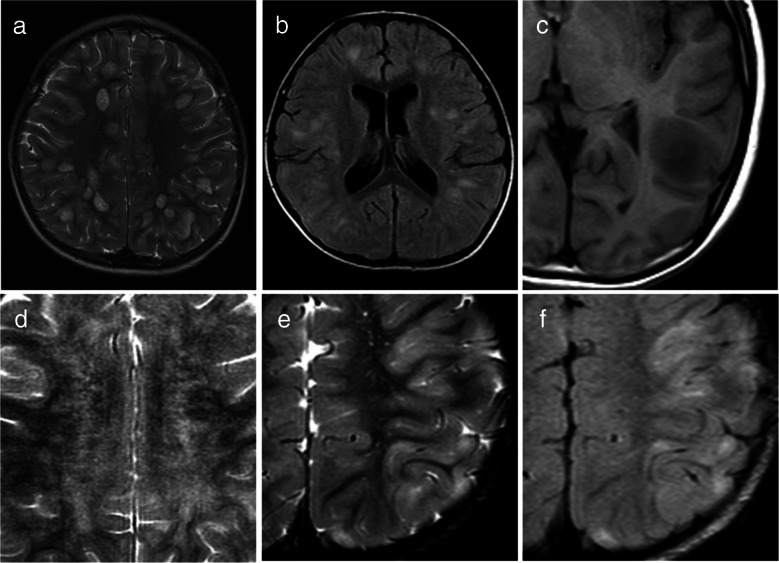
Representative magnetic resonance imaging features considered atypical for acute disseminated encephalomyelitis. a Axial T2-weighted image in a 5-year-old boy with myelin oligodendrocyte glycoprotein antibody-associated acute disseminated encephalomyelitis (MOG-ADEM) shows well-demarcated lesions predominantly involving subcortical white matter. b Axial fluid-attenuated inversion recovery image in a 5-year-old girl with MOG-ADEM shows small (< 2 cm) lesions. c Axial T1-weighted image in an 11-year-old girl with MOG-ADEM shows a T1-hypointense lesion (*arrow*) compared to gray matter. d Axial T2-weighted image in a 2-year-old girl with MOG-ADEM shows white matter lesions oriented perpendicular to the ventricles (*arrows*), appearing as laterally extending striations. e, f Axial T2-weighted (e) and fluid-attenuated inversion recovery (f) images in a 3-year-old boy with MOG-ADEM show cortical lesions (*arrows*)
